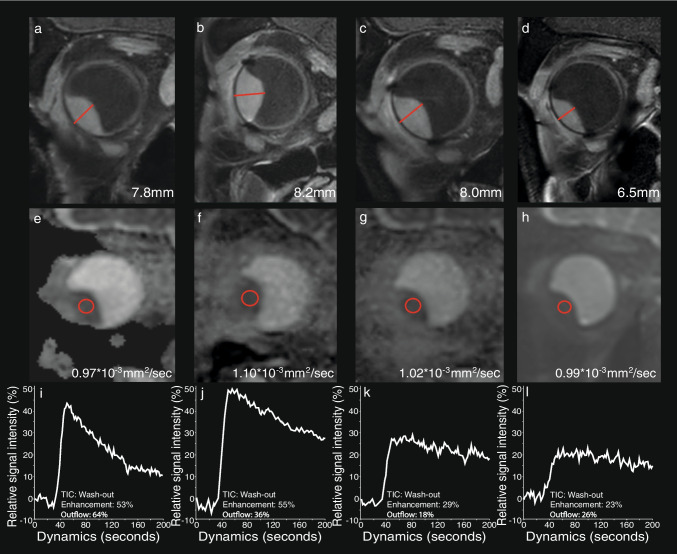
MRI of a UM of the right eye (a, e, i) before, and (b, f, j) 2, (c, g, k) 5 and (d, h, l) 11 months after PBT. Pseudo progression of the UM 2 months after radiotherapy: slight increase in size, indicated by red lines (a–d), but what seems a more favourable TIC in terms of perfusion outflow reduction at DCE MR Perfusion. a–d Coronal oblique MS TSE contrast-enhanced T1 with fat signal suppression. Slight increase in size of the UM 2 months after radiotherapy followed by a slow progressive decrease of its size. e–h Coronal oblique ADC. Slight increase of the UM ADC value at 2 months which remained stable. i–l DCE TIC. Favourable perfusion characteristics after PBT, with progressive smaller peak intensity and outflow percentage. ROIs used to derive the ADC values are delineated in red circles
